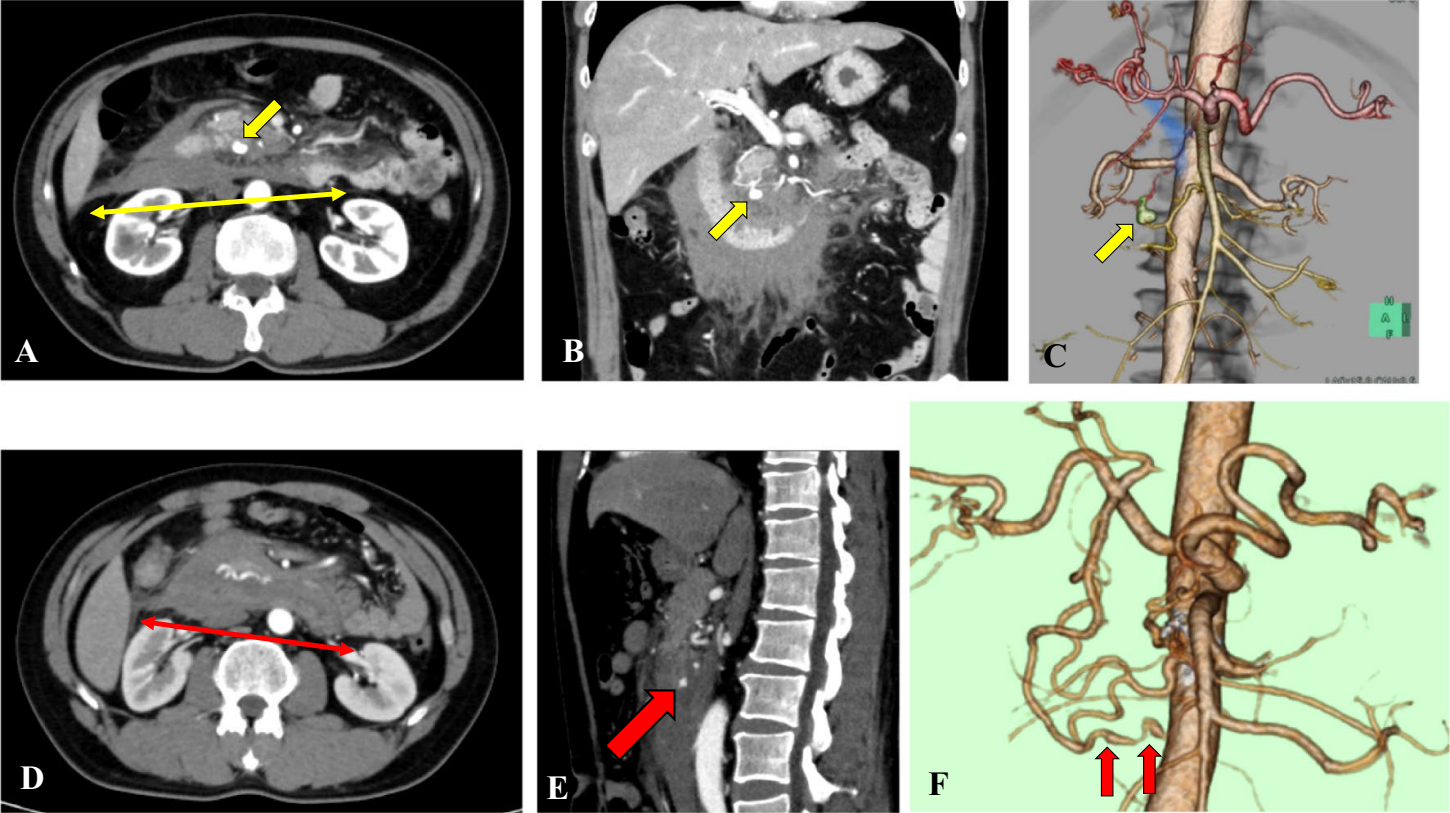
Representative computed tomography (CT) images of retroperitoneal hemorrhage in patients with COVID-19. A–C Axial, coronal and 3D CT images from a 56-year-old patient show evidence of retroperitoneal hemorrhage (thin yellow double-headed arrow) with pseudoaneurysm of the inferior pancreaticoduodenal artery (yellow arrows). D–F Axial, sagittal and 3D CT images from a 41-year-old patient show evidence of retroperitoneal hemorrhage (thin red double-headed arrow, D) with pseudoaneurysm of the anterior inferior pancreaticoduodenal artery (red arrows)
